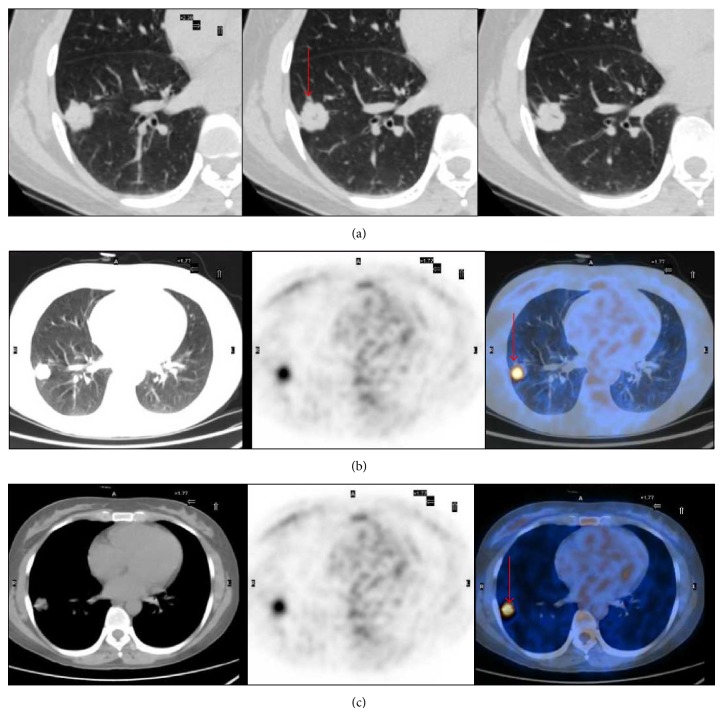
A 47-year-old woman with an adenocarcinoma lesion with BAC features (red arrow). (a) Axial thin section CT image revealed a 2.2 cm solid nodule (arrow) in right lower lobe. (b, c) ^18^F-FDG PET/CT was positive for the lesion, and the maximum standardized uptake value = 5.66.
